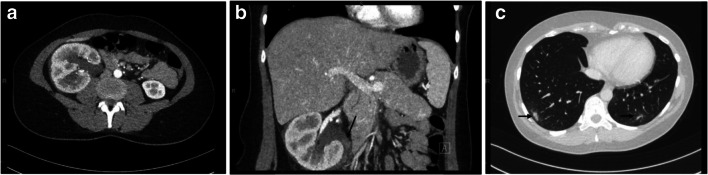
Contrast-enhanced CT scan at presentation, 100 seconds after intravenous contrast. a, b Microcalcifications and severe hydronephrosis of the right kidney. A dense mass of 75 HU, corresponding to the density of a blood clot, can be seen at the transition of the pyelum to the proximal ureter and is indicated by an arrow. A slight layer of fluid around the kidney can be seen and is suggestive of inflammation. There is a delayed arrival of contrast to the right kidney compared with the left side. In a later phase of the scan, retention of contrast in the right pyelum with delayed drainage was observed. c Ground glass abnormalities (arrows) in the peripheral basal lung areas which were deemed to be remnants of COVID-19
